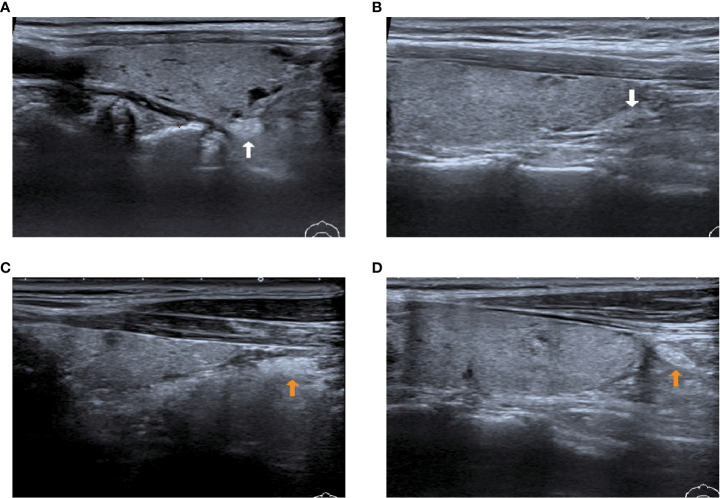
Two types of IPG observed by preoperative ultrasound images. (A, B): White arrow shows type I PG, where the PGs are in close contact with the thyroid gland; (C, D): Yellow arrow shows type II PG, where the PGs are free of the thyroid gland and not in close contact with the thyroid gland.
